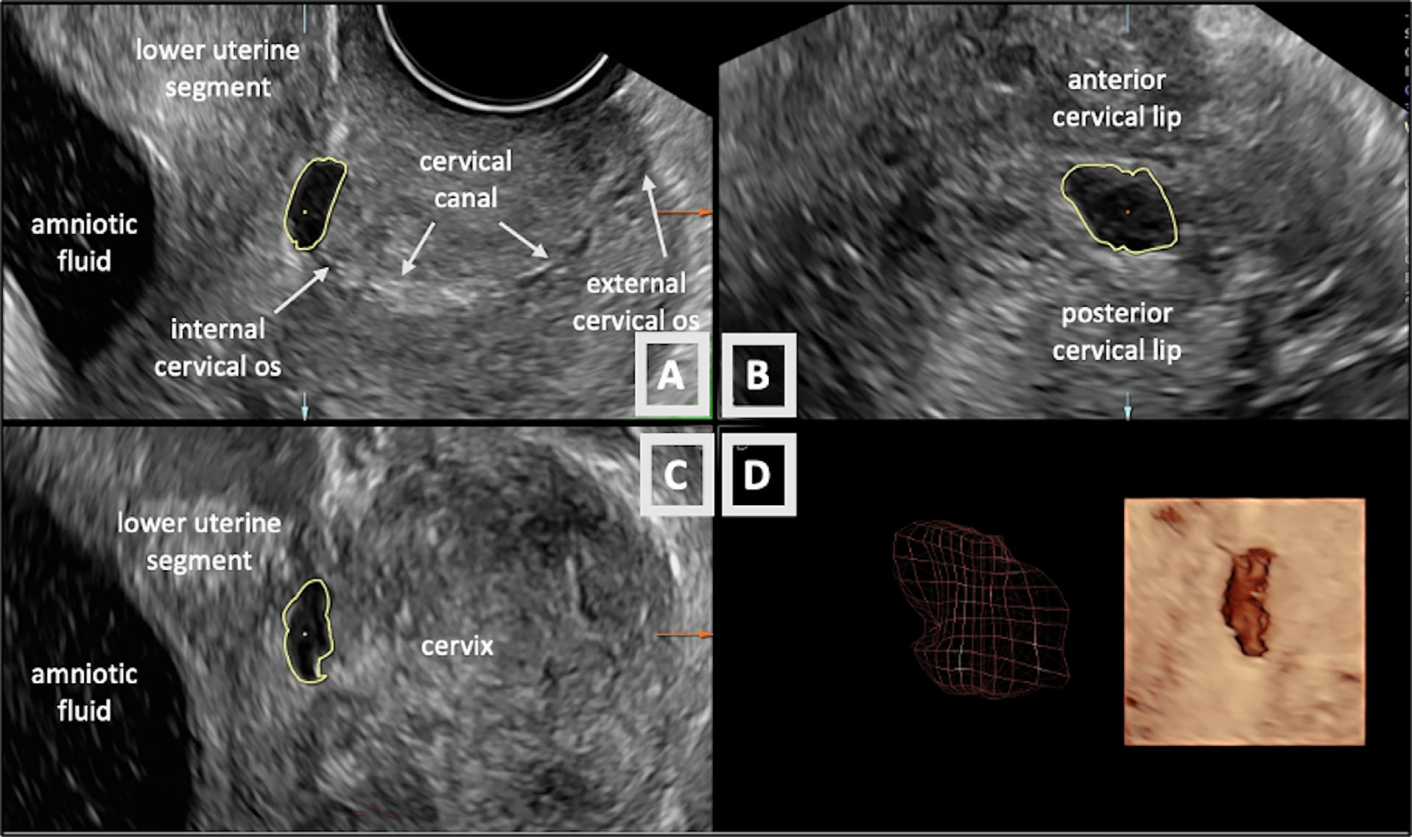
Manipulation of 3‐dimensional ultrasound volume of the lower uterine segment and cervix in sagittal (A), transverse (B), and coronal (C) planes using VOCAL. Three‐dimensional geometric reconstructions of a cesarean scar niche (D) measuring 209 mm^3^.
